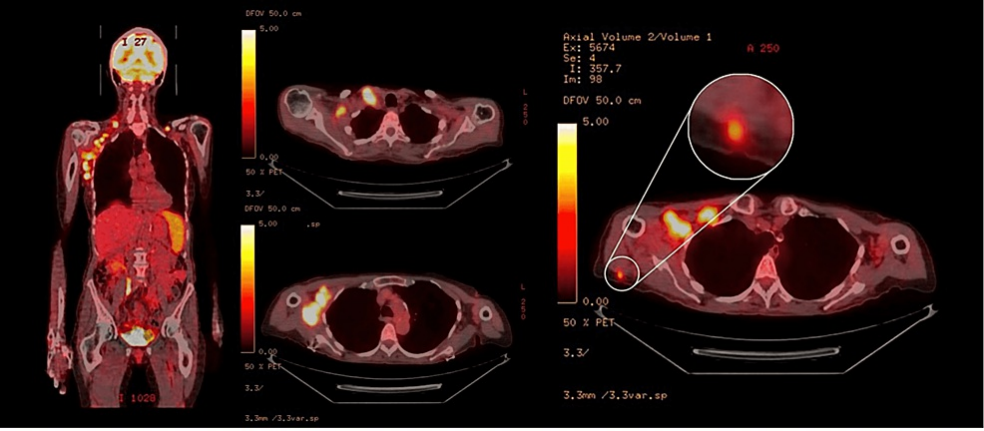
Fused images of an F-18 FDG PET-CT scan Fused images of an F-18 FDG PET-CT scan show numerous hypermetabolic lymph nodes in the right axilla. The spleen is diffusely hypermetabolic, concerning lymphomatous involvement. A hypermetabolic subcutaneous nodule in the right shoulder may also represent extranodal involvement. F-18 FDG: F-18 Fluorodeoxyglucose; PET/CT: Positron emission tomography-computed tomography
